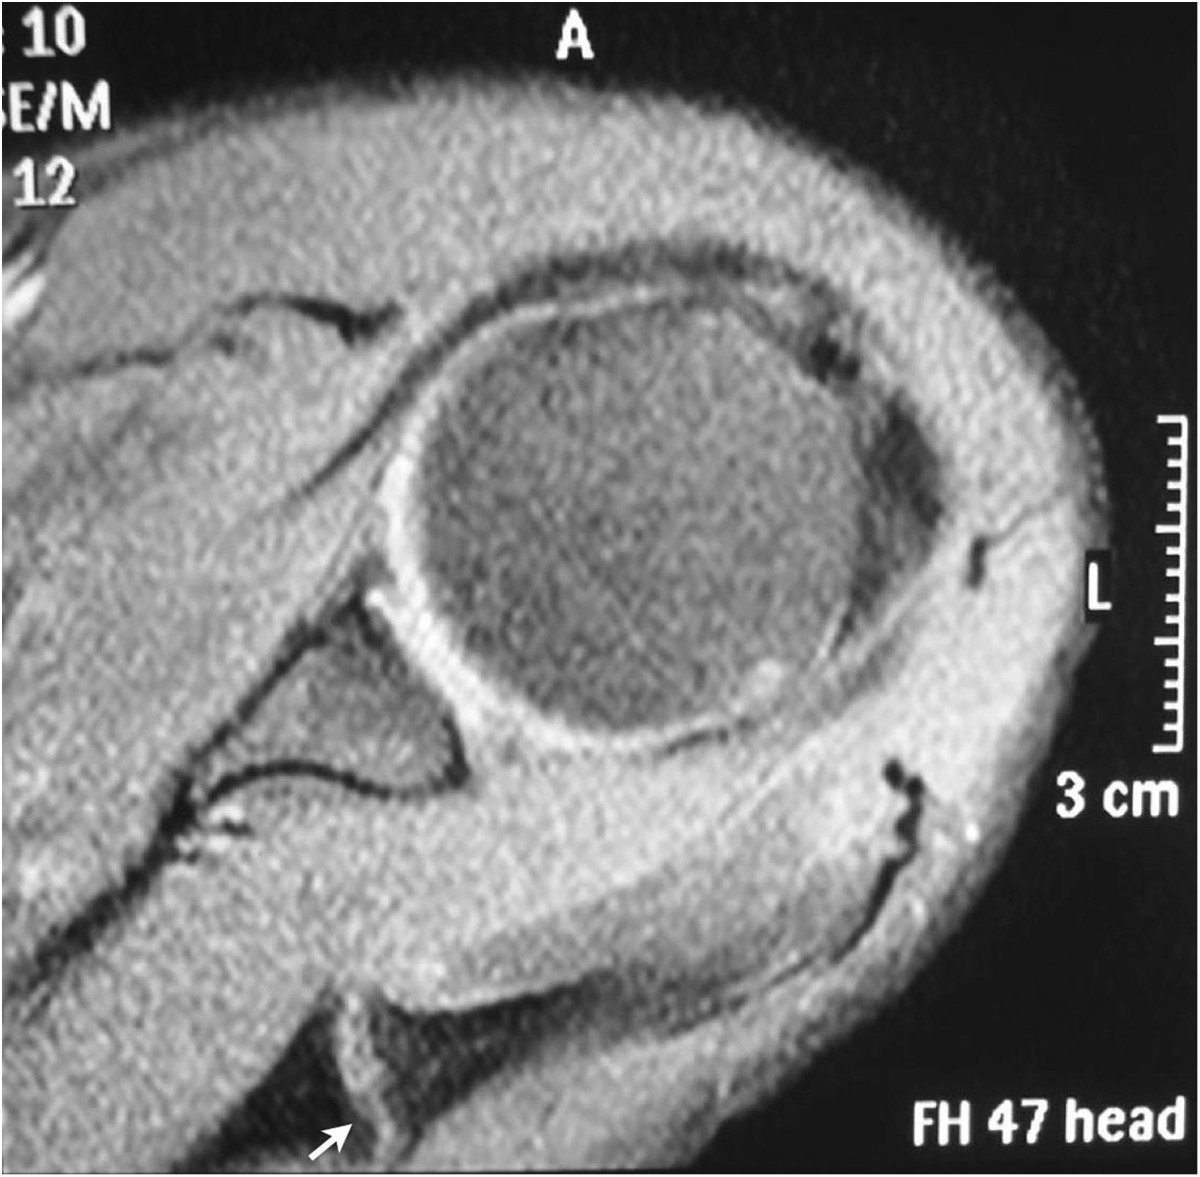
Preoperative magnetic resonance imaging (MRI). An axial section of the T2-weighted MRI demonstrating a hypertrophic pseudarthrosis of the fracture of the base of the acromion (arrow).
